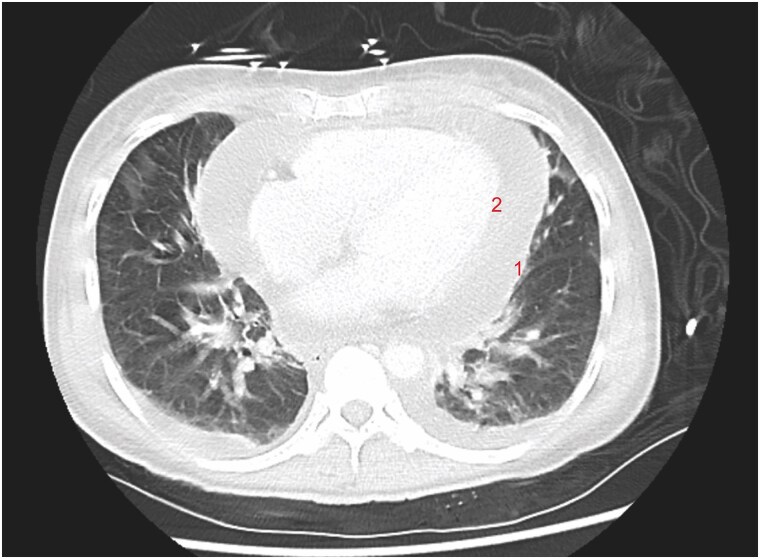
Axial computed tomography scan showing a large circumferential pericardial effusion. The effusion is located between the parietal pericardium (1) and visceral pericardium (2).
